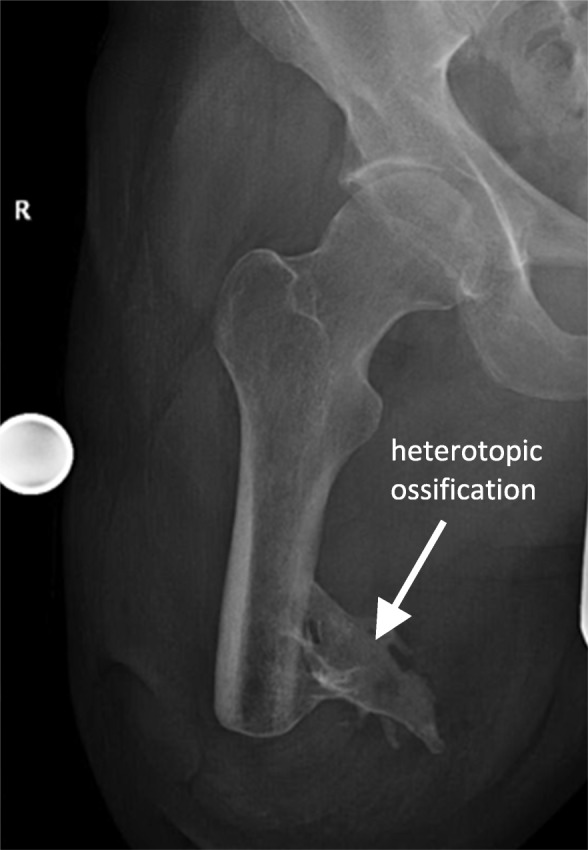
Preoperative x-ray with a 30-mm reference ball to plan an EEP treatment. Large ossification depicted at the distal medial femur
